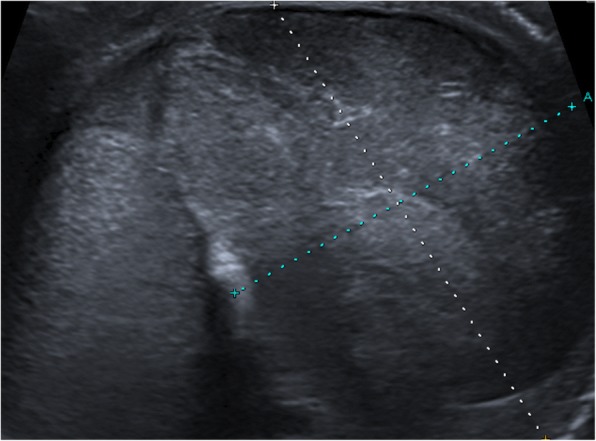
Ultrasound of the same testis left, after presentation at the ER. Lateral of the testis, a large hypo echogenic, non-vascular mass of 39×29mm is visible
